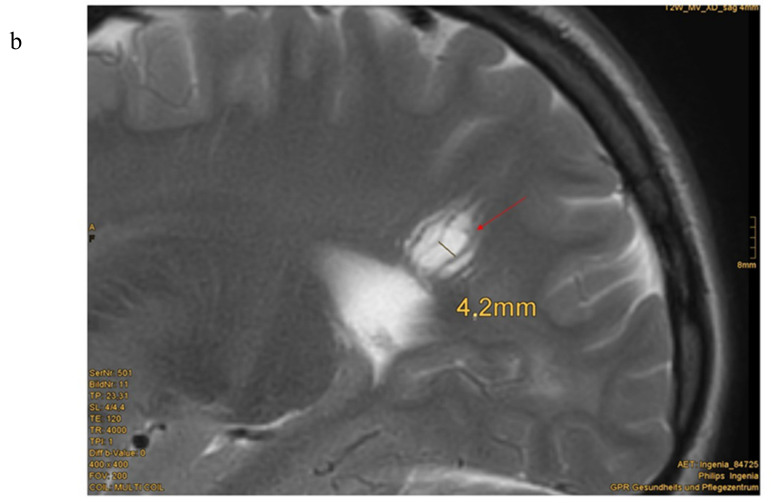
Enlarged perivascular spaces (EPVS) in cerebral MRI (a) Patient 15: 4.5 year-old boy, T2w-image, EPVS 2 mm diameter [Courtesy Kinderkrankenhaus Kliniken der Stadt Köln]. (b) Patient 23: 12.5 year old girl, T2 weighted image, sagittal view, pronounced EPVS up to 3 mm diameter [Courtesy Dr. A. Wieschen, Institut für Radiologie und Nuklearmedizin, GPR Klinikum Rüsselsheim].
